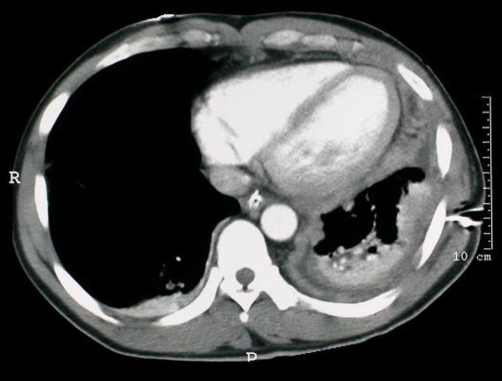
CT scan of the chest shows extensive left basilar pleural disease with loss of lung volume, nodular left pleural thickening and a nodular pericardium. Nodules in the right lung are consistent with metastatic disease.
